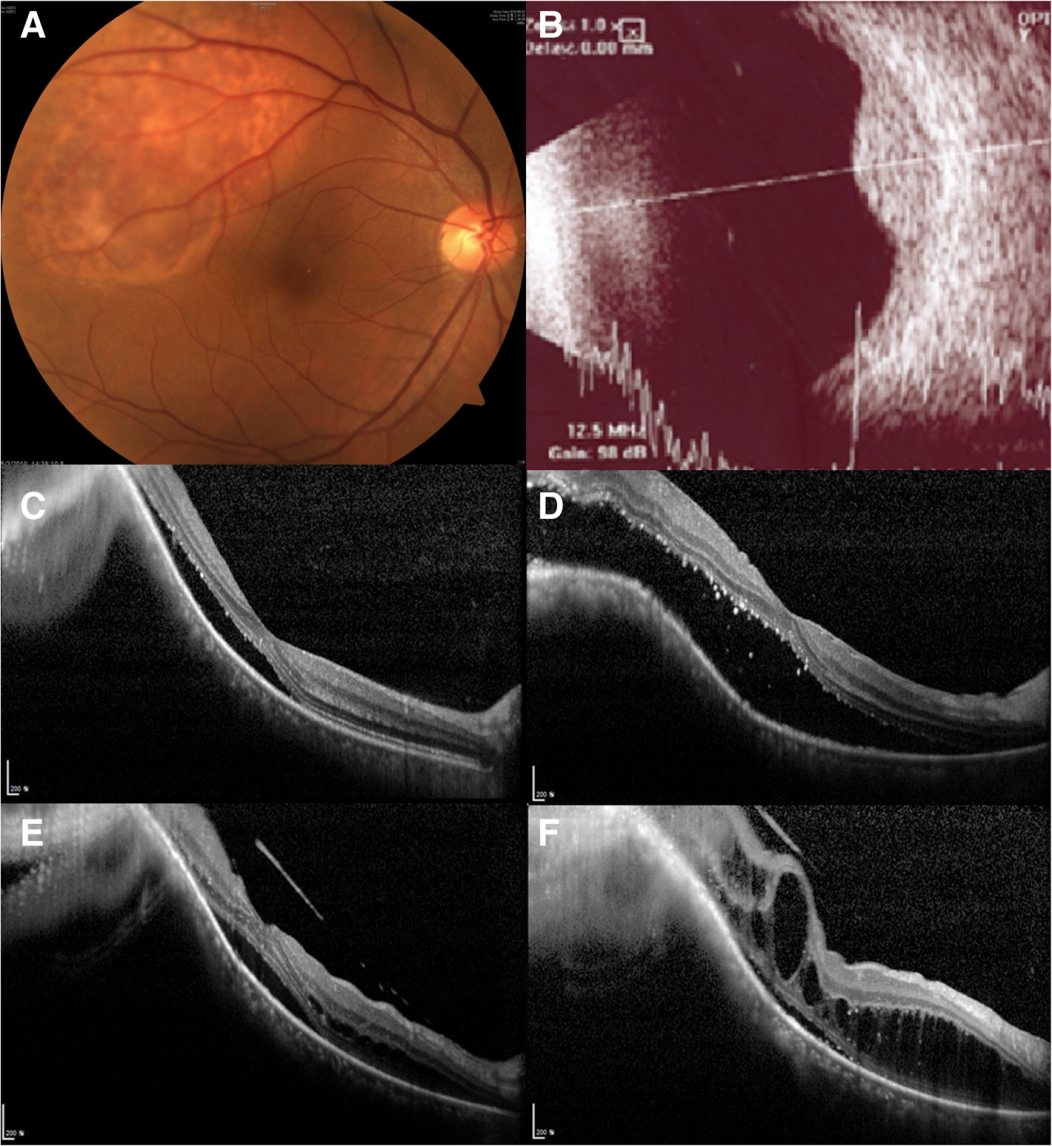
A representative case of circumscribed choroidal hemangioma showing the change in retinal fluid over time. A 48-year-old woman (Case 7) presented with blurred vision of the right eye. Her initial best corrected visual acuity (BCVA) was 0.63. (a) On fundus photography, an orange and red-colored subretinal mass was observed supero-temporally to the macula. (b) B-scan ultrasonography showed a hyperreflective mass with a height of 4.77 mm and base diameter of 11.07 mm. (c) Shallow subretinal fluid involving the fovea was observed along with elevation of the choroid by a mass lesion on optical coherence tomography. (d) During the first 6 months, despite a single intravitreal bevacizumab (IVB) injection, the subretinal fluid (SRF) increased. Thereafter, a second IVB injection and single session of transpupillary thermotherapy (TTT) were performed; however, there was no significant improvement in therapeutic effect. Subsequently, a single session of photodynamic therapy (PDT) and enhanced PDT were performed and the SRF completely resolved. However, the subsequent BCVA was 0.04. (e) After 2 years of follow-up, SRF and intraretinal fluid then developed. (f) Six months later, an advanced cystoid macular edema pattern was observed
